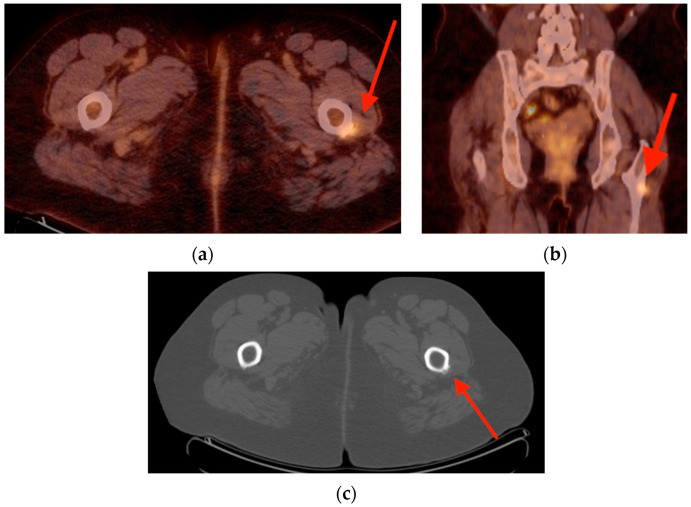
Axial (a) and coronal (b) FDG PET/CT images from a 63-year-old woman with head and neck adenocarcinoma show focal uptake in the region of the vastus lateralis origin, with SUVmax 3.7 (red arrows). (c) Plain CT images show periosteal changes consistent with enthesopathic changes of the vastus lateralis (red arrow). CT = computed tomography. FDG PET/CT = fluorine-18 fluorodeoxyglucose positron emission tomography/computed tomography. SUVmax = maximum standard uptake value.
